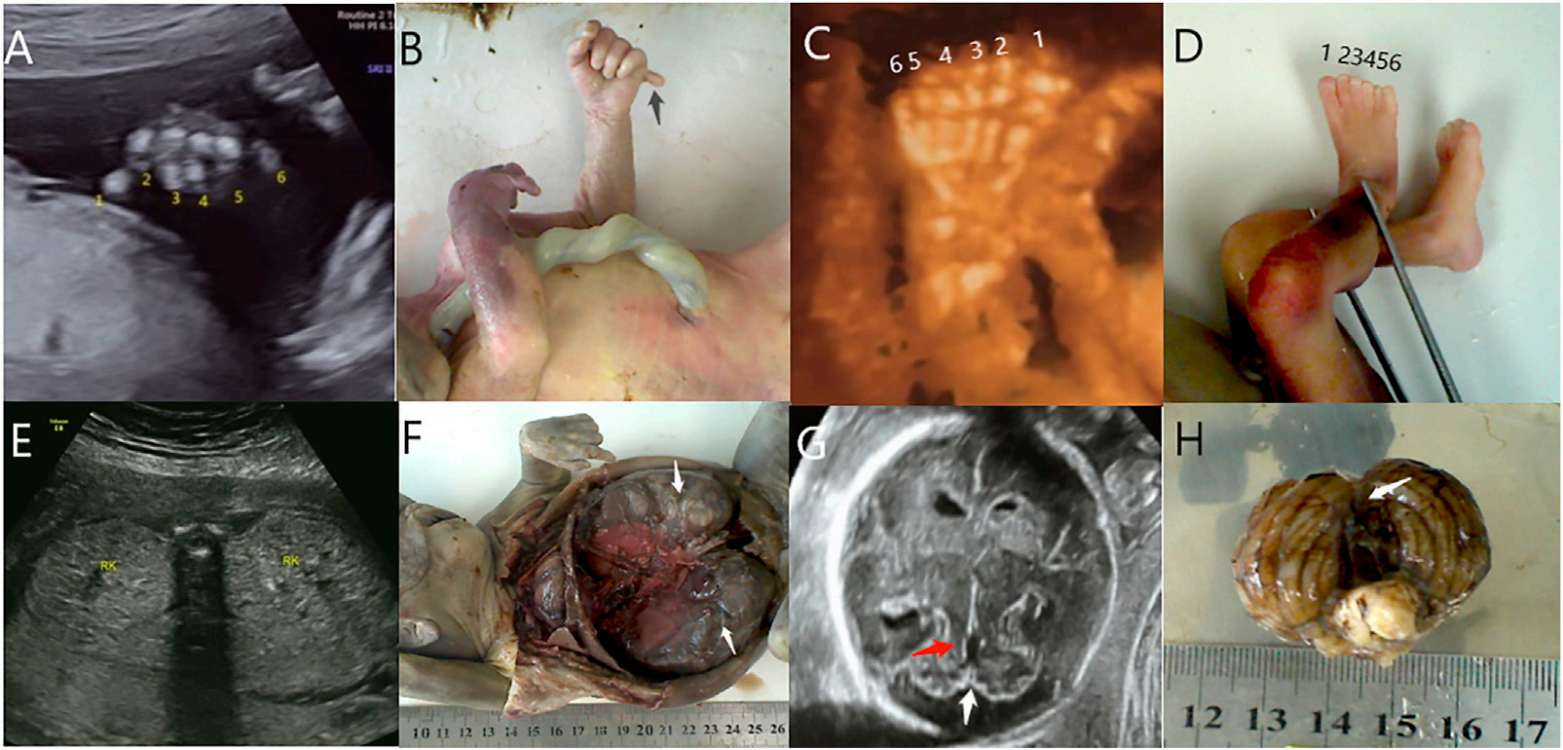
Polydactyly (black arrow) was detected by ultrasound (A) and autopsy (B) in case 1. Polydactyly was detected by ultrasound (C) and autopsy (D) in case 3. Polycystic kidneys (white arrow) were detected by ultrasound (E) and autopsy (F) in case 8. (G) The mild MTS (red arrow) and CV absence (white arrow) were detected by ultrasound in case 9. (H) CV absence (white arrow) was detected by an autopsy in case 9.
